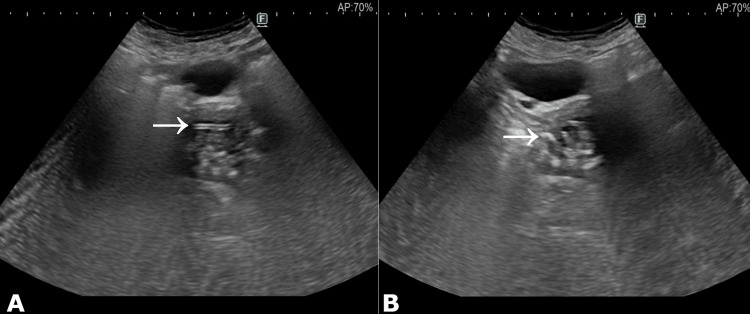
Transabdominal ultrasound images of the pelvis (A) Sagittal plane and (B) axial plane demonstrating an echogenic linear structure (arrows) with posterior acoustic shadowing within the endometrial cavity, consistent with an intrauterine device.
